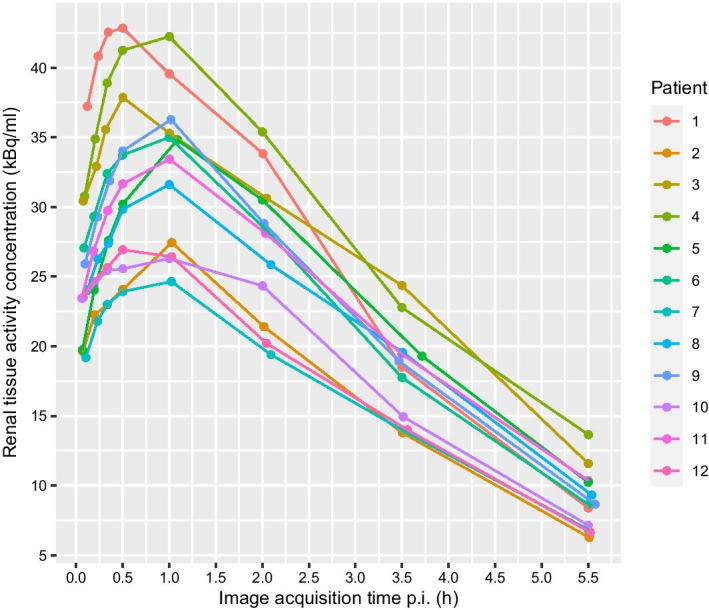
Renal tissue activity concentration at different time points after ^18^F-PSMA-1007 injection
